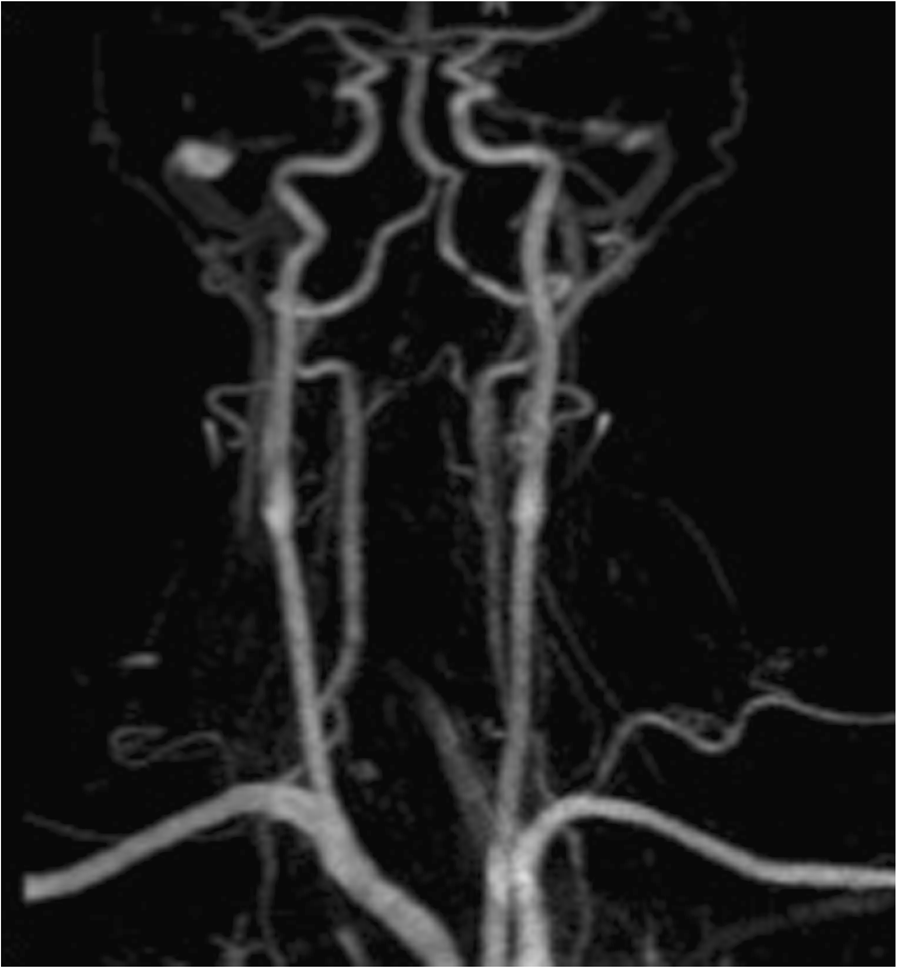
Magnetic resonance imaging angiography of the cervical vessels.
